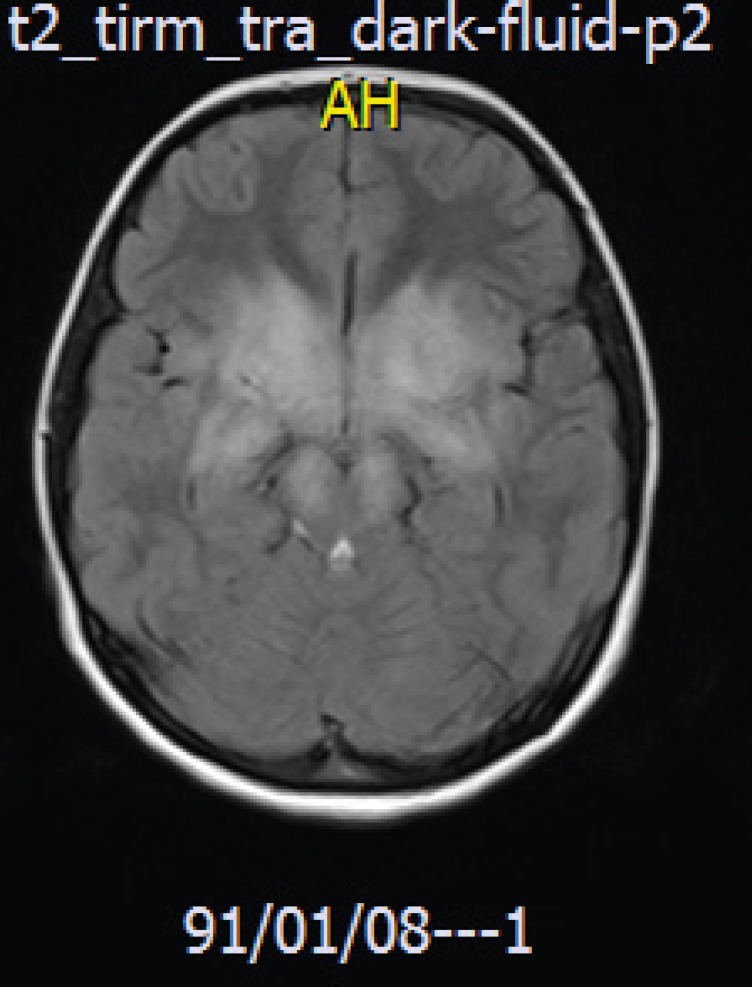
Brain MRI Flaire image showing hyperintensities in basal ganglias
